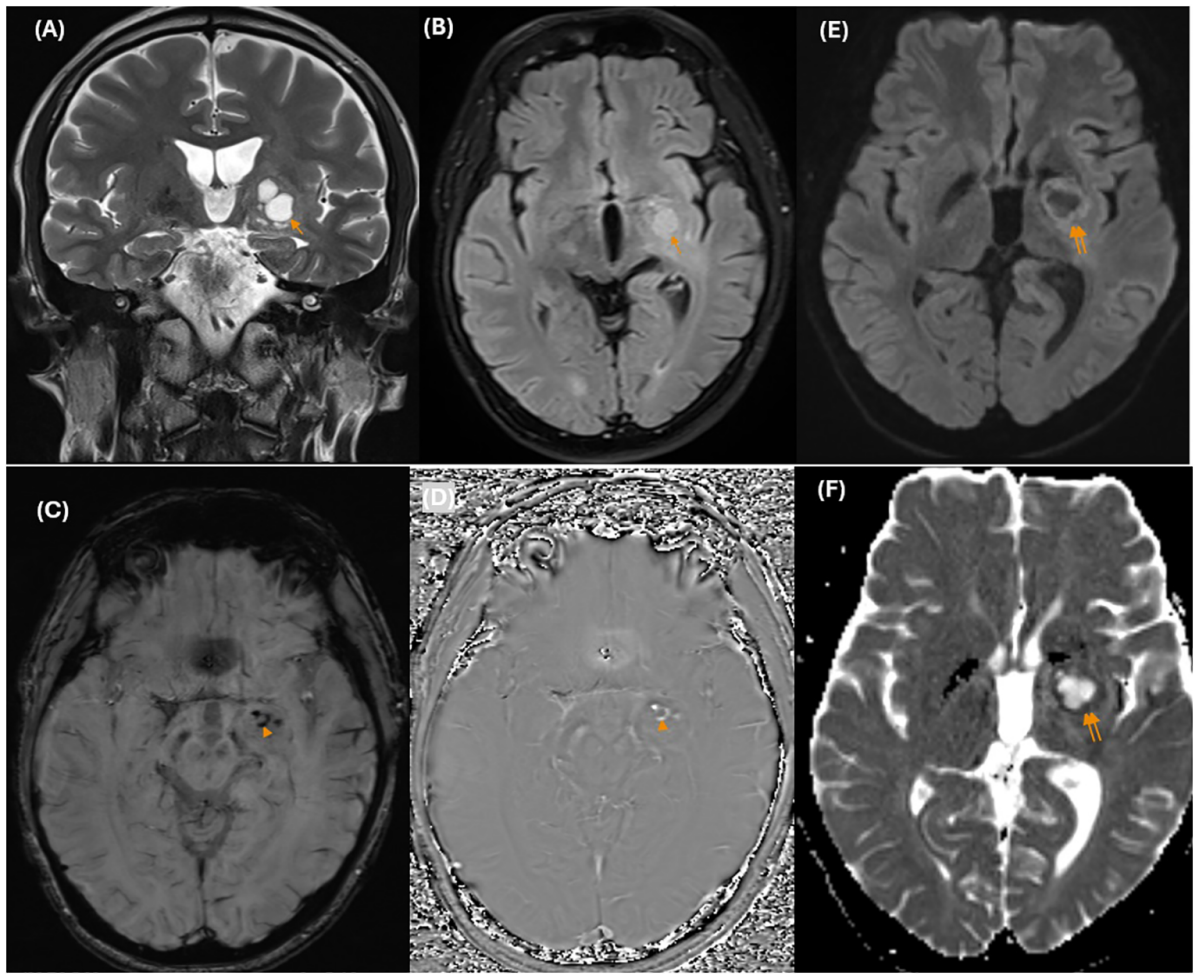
On conventional MR images, coronal T2-weighted images (A) show an ill-defined hyperintense mass centered on the left basal ganglia with cystic changes (arrow) that are not completely suppressed on FLAIR images (B). There are several hypointense dots (arrowhead) on the SWI images (C), and the corresponding hyperintensity on the phase image (D) represents hemorrhage. There was hyperintensity on DWI (E) and corresponding hypointensity on the ADC maps (F). *MR, magnetic resonance; T2W, T2 – Weighted; FLAIR, Fluid-Attenuated Inversion Recovery; SWI, Susceptibility weighted imaging; DWI, Diffusion-weighted imaging; ADC, Apparent diffusion coefficient.
